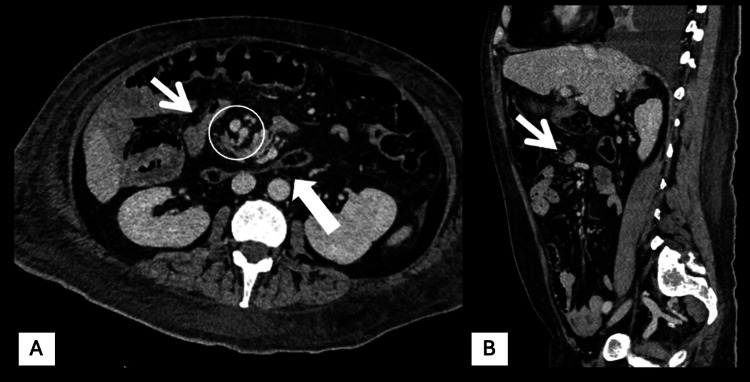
Duodenojejunal junction (A) Contrast-enhanced axial CT image in the venous phase demonstrating the duodenojejunal junction (thin arrow) located to the right of the superior mesenteric vessels (circle) (thick arrow: transverse colon). (B) Sagittal reconstruction confirming right-sided location of the duodenojejunal junction (thin arrow).
